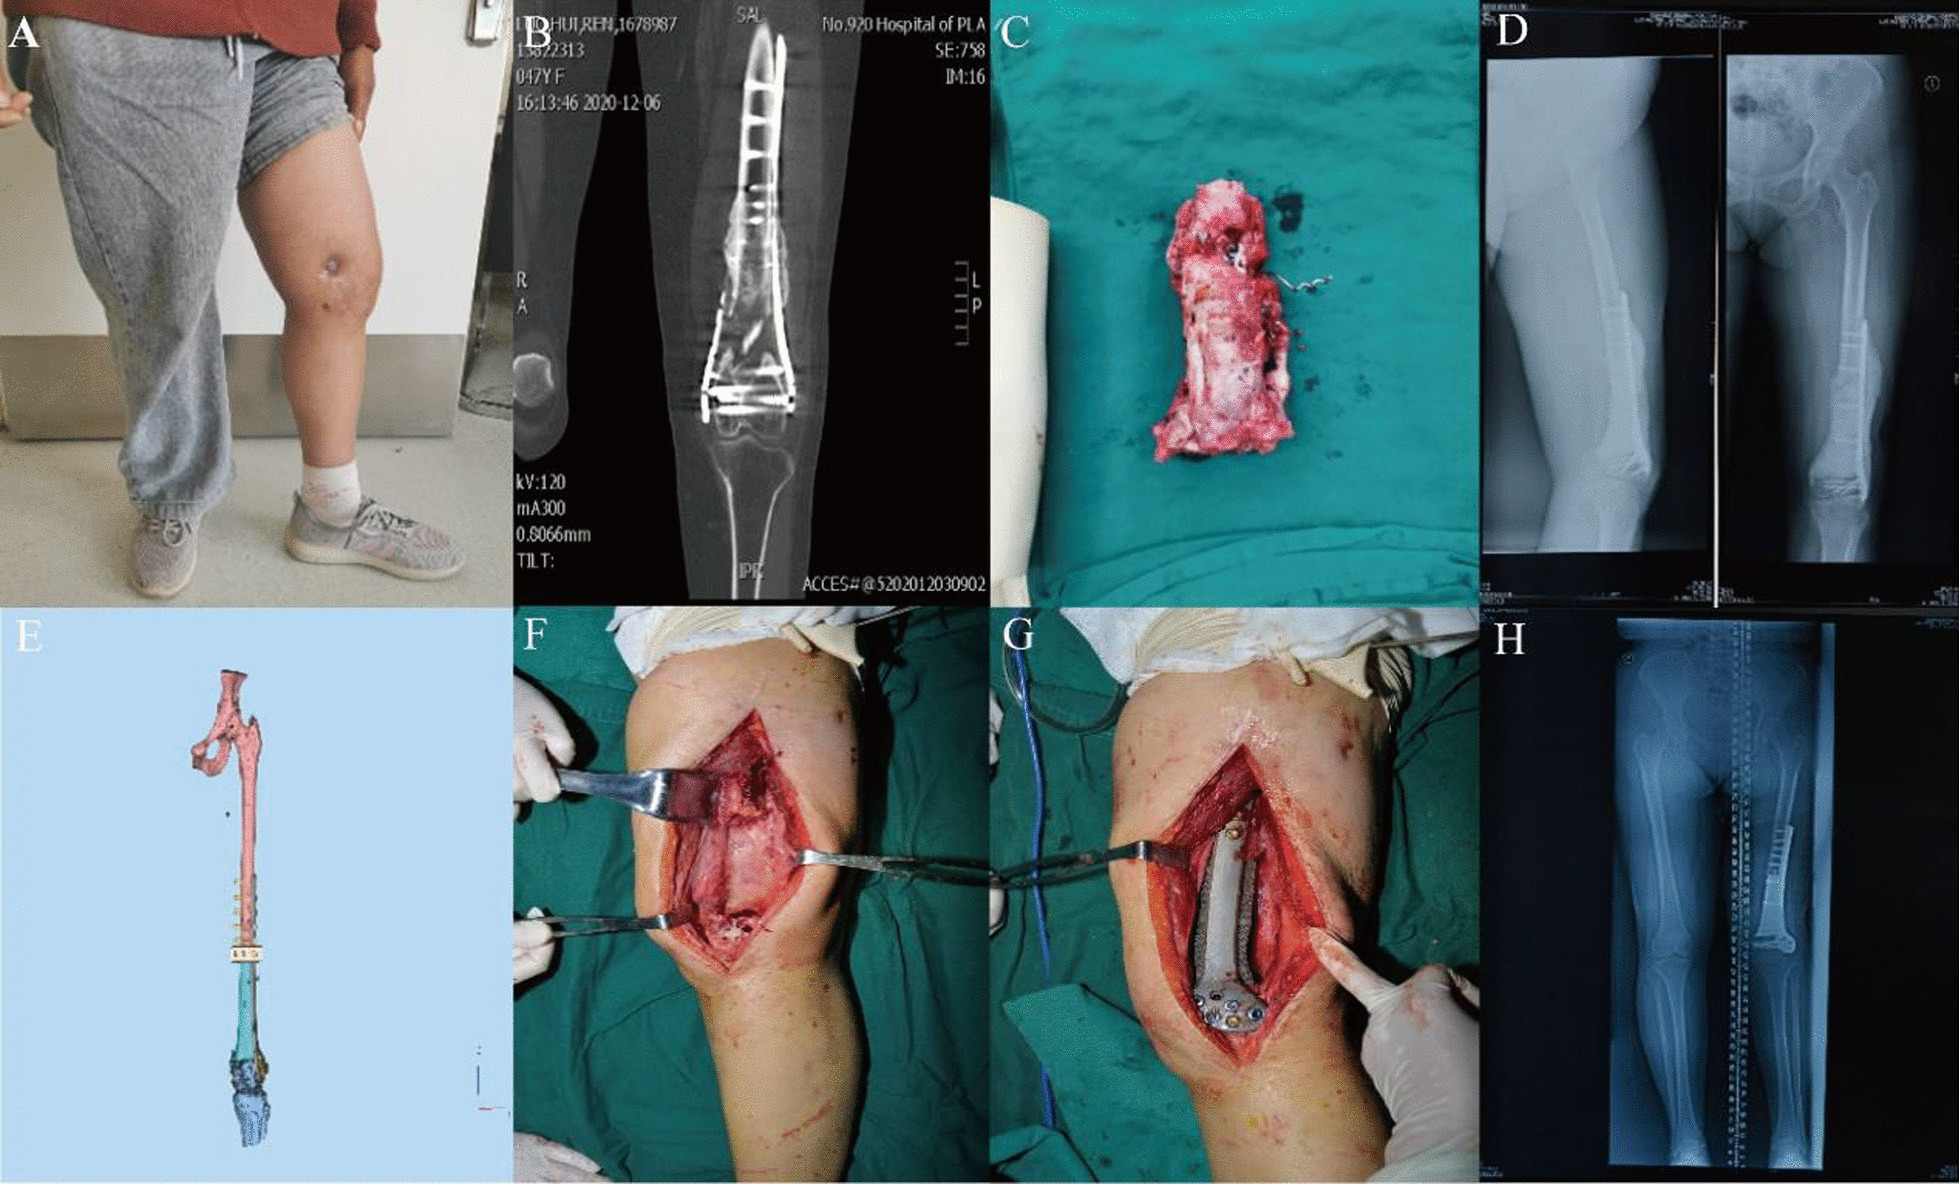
A typical case. A A 26-year-old female who suffered from comminuted femoral fracture in the distal section, two anatomical plates were used in the preliminary surgery, osteomyelitis occurred 3 months later with a sinus tract formation at inner side of thigh; B CT scan shows infective osteonecrosis in the distal section of left femur; C Removed the internal fixation and necrosis bone, the length of infective bone was approximate 15.9 cm; D Antibiotics-loaded bone cement spacer implanted into the defect section after debridement, combined with temporary fixation by a locking titanium plate on the lateral side of femur; E the photograph of real product, the prominent structure could insert into the medullary cavity to increase the contact area between the bone and prosthesis. F In the stage 2, remove all implantation in stage 1 and protected the self-induced membrane; G filled the segmental bone defect area with the scaffolds; H 13 months later, radiological tests showed satisfactory osseointegration of the scaffolds and stability fixation
